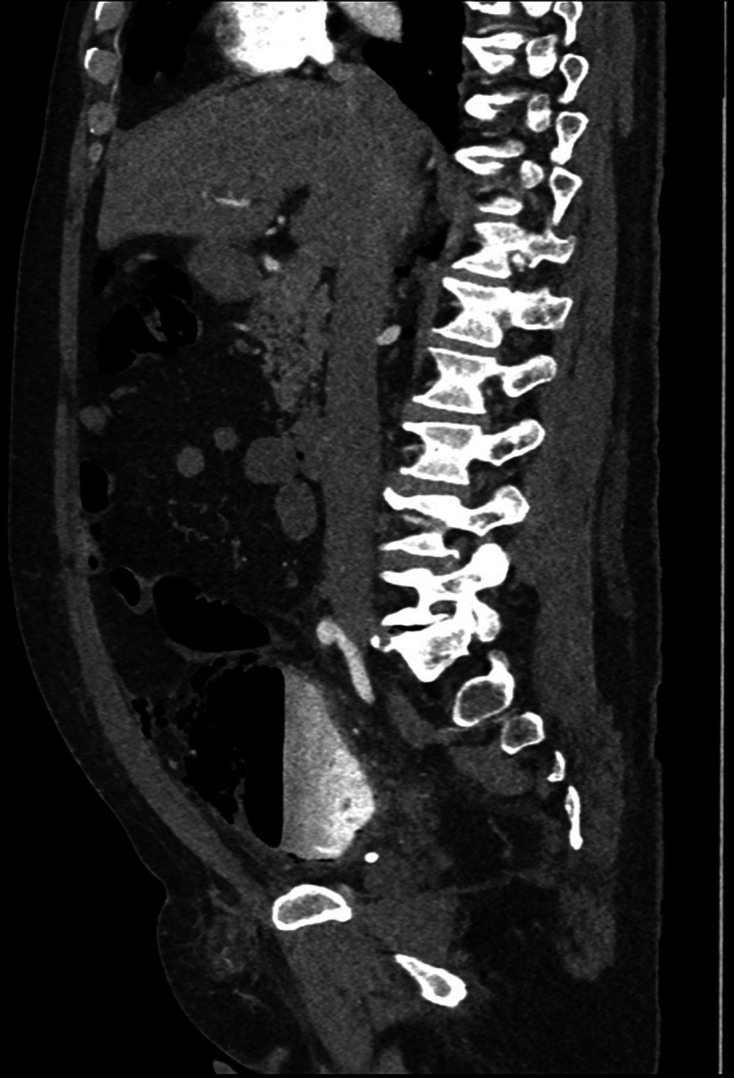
First sagittal delayed phase CT (day 3 of admission), on soft tissue window
